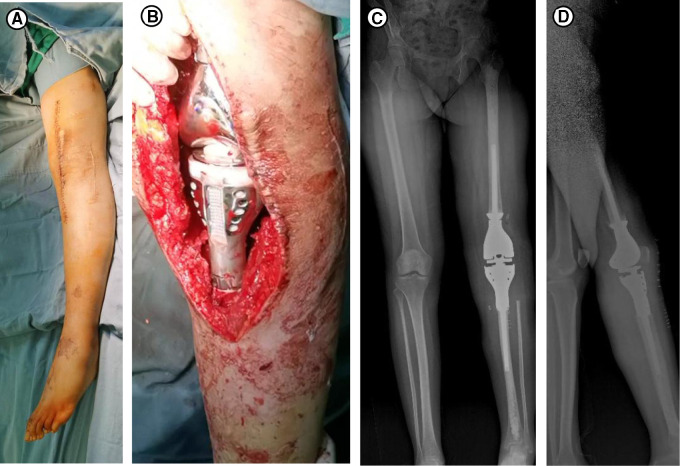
(A) Disinfection for the second operation. (B) Prosthesis of the left knee joint. (C,D) X-rays taken 2 weeks after the second surgery, (C) is anteroposterior and (D) is lateral.
